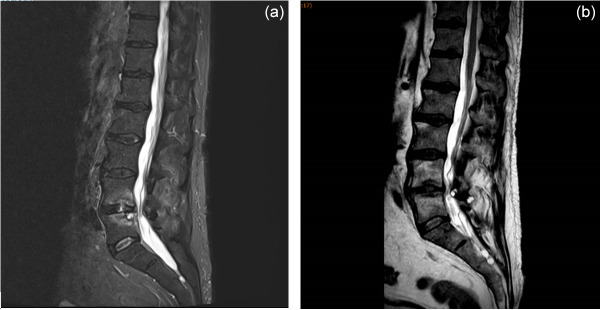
MRI sagittal planes of L4–L5 spondylodiskitis showing (a) bone erosions and epiduritis at diagnosis and (b) the resolution of lesions 5 months after the end of rezafungin treatment.
